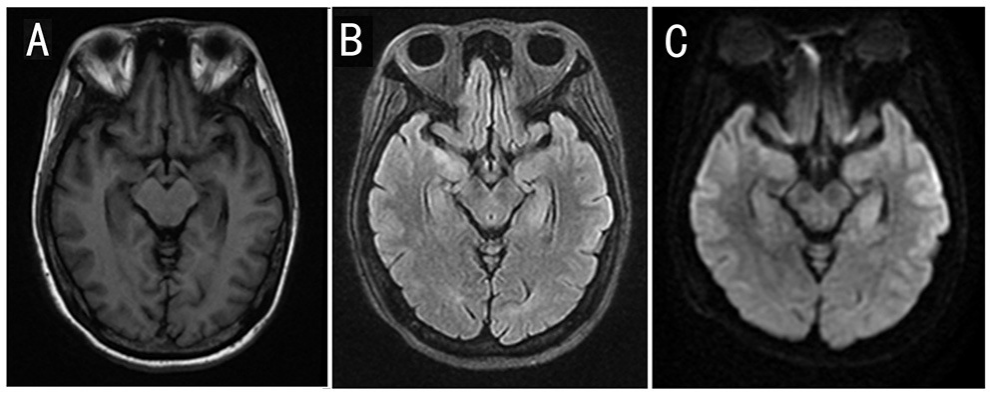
Cranial MRI results of the patient. Cranial MRI showed an increased signal intensity in the mesial temporal lobes and the hippocampus on T2 FLAIR and diffusion-weighted images. (A) T1-weighted image. (B) T2 FLAIR image. (C) Diffusion-weighted image.
